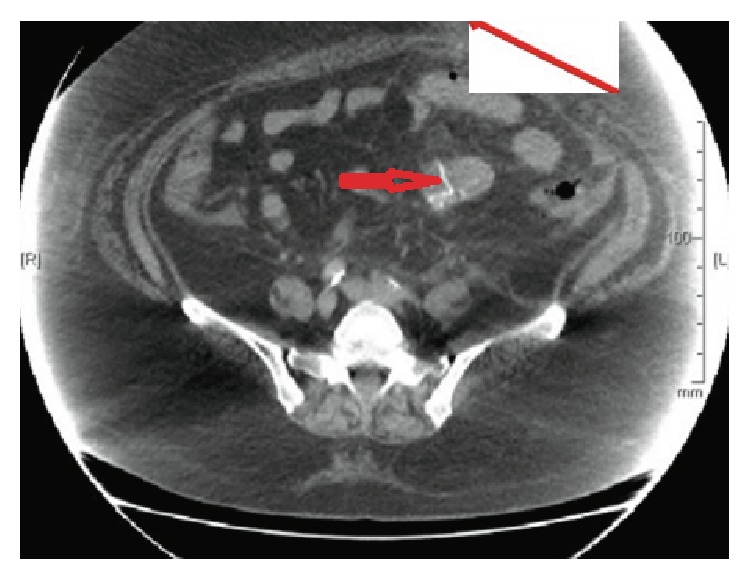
CT contrast scan depicting bowel anastomosis after 1st surgery (short red arrow).
